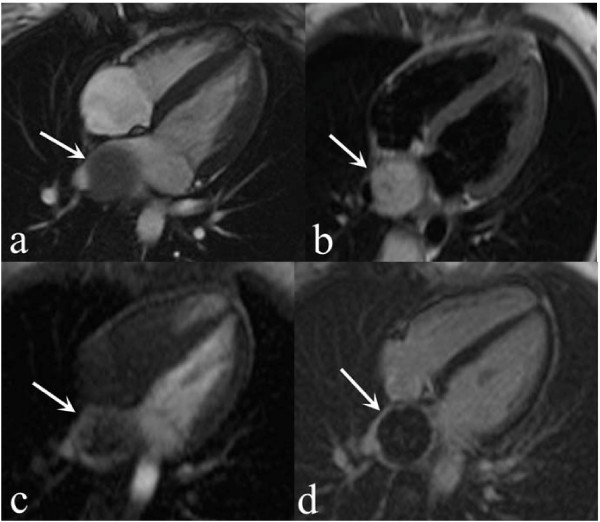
Horizontal long axis CMR images demonstrate a round, well defined posterior mediastinal mass with smooth extrinsic compression of left atrial wall which is hypo-intense on breath-hold Steady State Free Precession (SSFP) cine CMR (a, arrow), and shows homogenously high signal on T2-weighted dark blood turbo spin-echo image (b, arrow). T1-weighted inversion recovery gradient-echo myocardial perfusion imaging demonstrates heterogeneous, mostly peripheral, dynamic contrast filling of the mass suggesting vascularity (c, arrow), and T1-weighted, fat-saturated, inversion recovery late gadolinium enhancement image shows peripheral rim enhancement with no evidence of central enhancement suggestive of central tissue necrosis (d, arrow).
